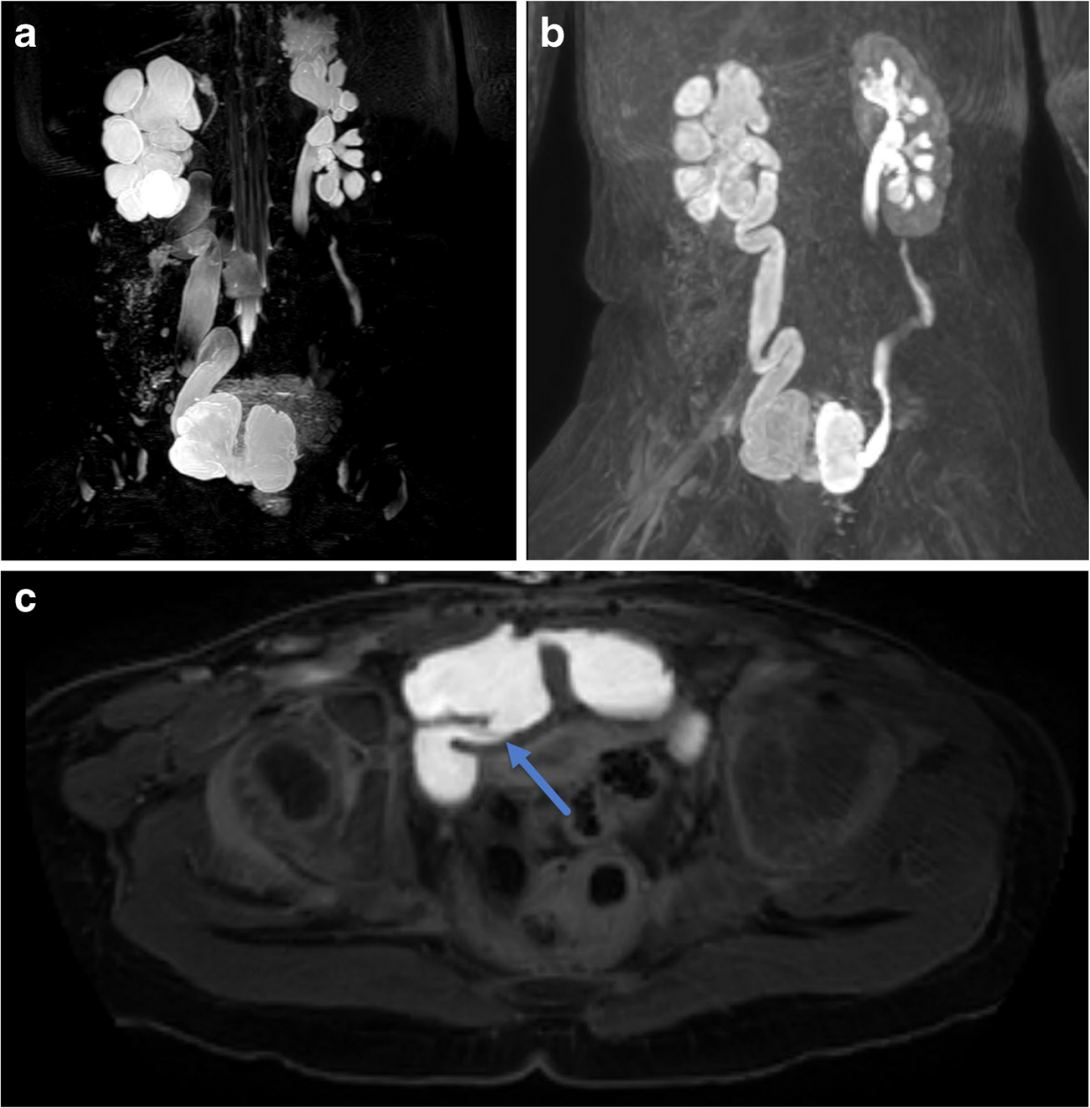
Three-year-old born premature at 27 weeks gestational age with history of bladder exstrophy post-repair with neobladder creation and vesicostomy. a MIP reconstruction of 3D T2-weighted fast spin-echo image shows marked right pelvocaliectasis and a dilated, tortuous right ureter. There is mild left hydroureteronephrosis. The neobladder has a bilobed appearance. b MIP reconstruction of 3D post-contrast excretory phase imaging shows symmetric excretion of contrast into both renal collection systems and ureters which freely flows into the bilobed neobladder. c Axial post-contrast excretory phase image shows a patent right ureterovesical junction (arrow)
